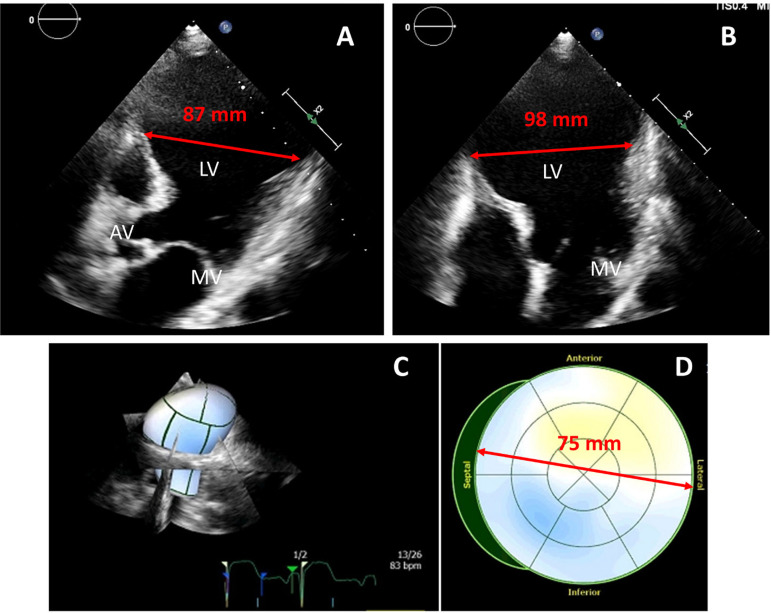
Preoperative transthoracic echocardiography with depiction of large left ventricular apex aneurysms in patients prior to left ventricular assist device implantation with concomitant Dor plasty. Five-chamber view of patient n. 1 with a left ventricular apex aneurysm of 87 mm (A), fourchamber view of patient n. 5 with a left ventricular apex aneurysm of 98 mm and impending rupture (B), and 3D left ventricular apex reconstruction of patient n. 4 with a septolateral diameter of 75 mm (C, D). AV=aortic valve; LV=left ventricle; MV=mitral valve
